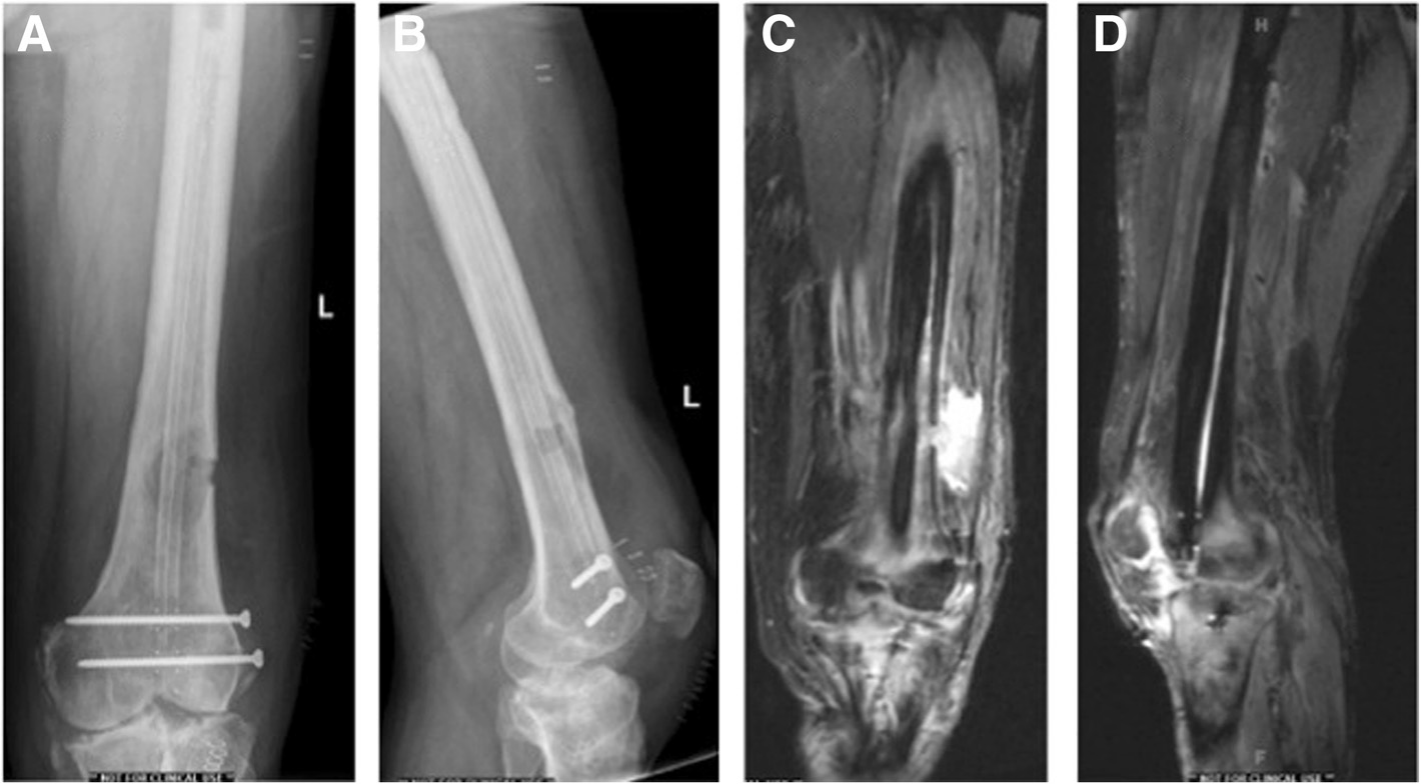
Post-operative images. (A) and (B) are AP and lateral postoperative X-rays of the distal femur demonstrating the radiolucent antibiotic impregnated cement carbon fiber interlocked nail with titanium screws. (C) and (D) are coronal and sagittal MRI STIR sequences demonstrating the absence of artifact This should allow for closer monitoring of the inflammation and disease progression.
